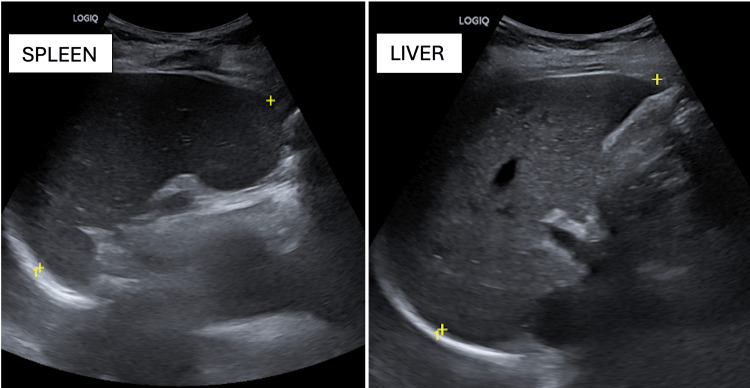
An ultrasonogram of the abdomen without hepatosplenomegaly
